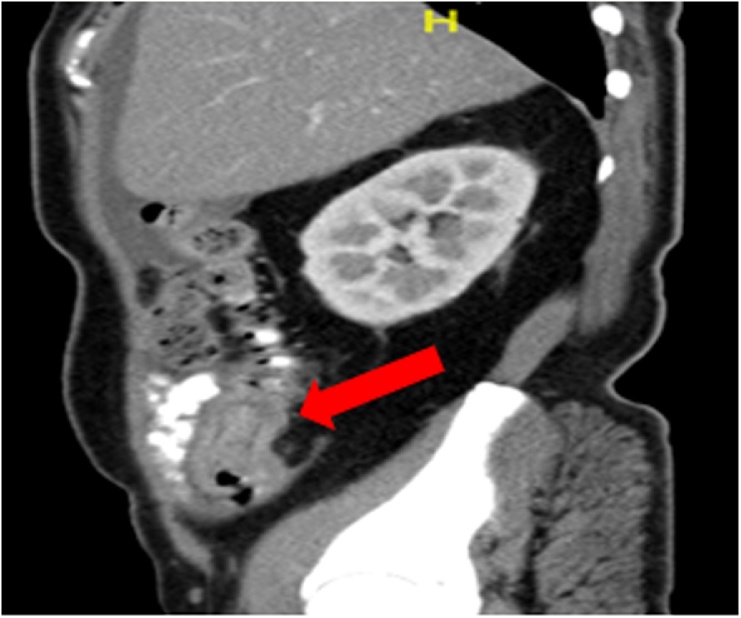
Sagittal section of the abdominal CT scan showing an entrapped bowel segment within an edematous outer bowel loop, marked by a red arrow. The proximal small bowel was not dilated.
